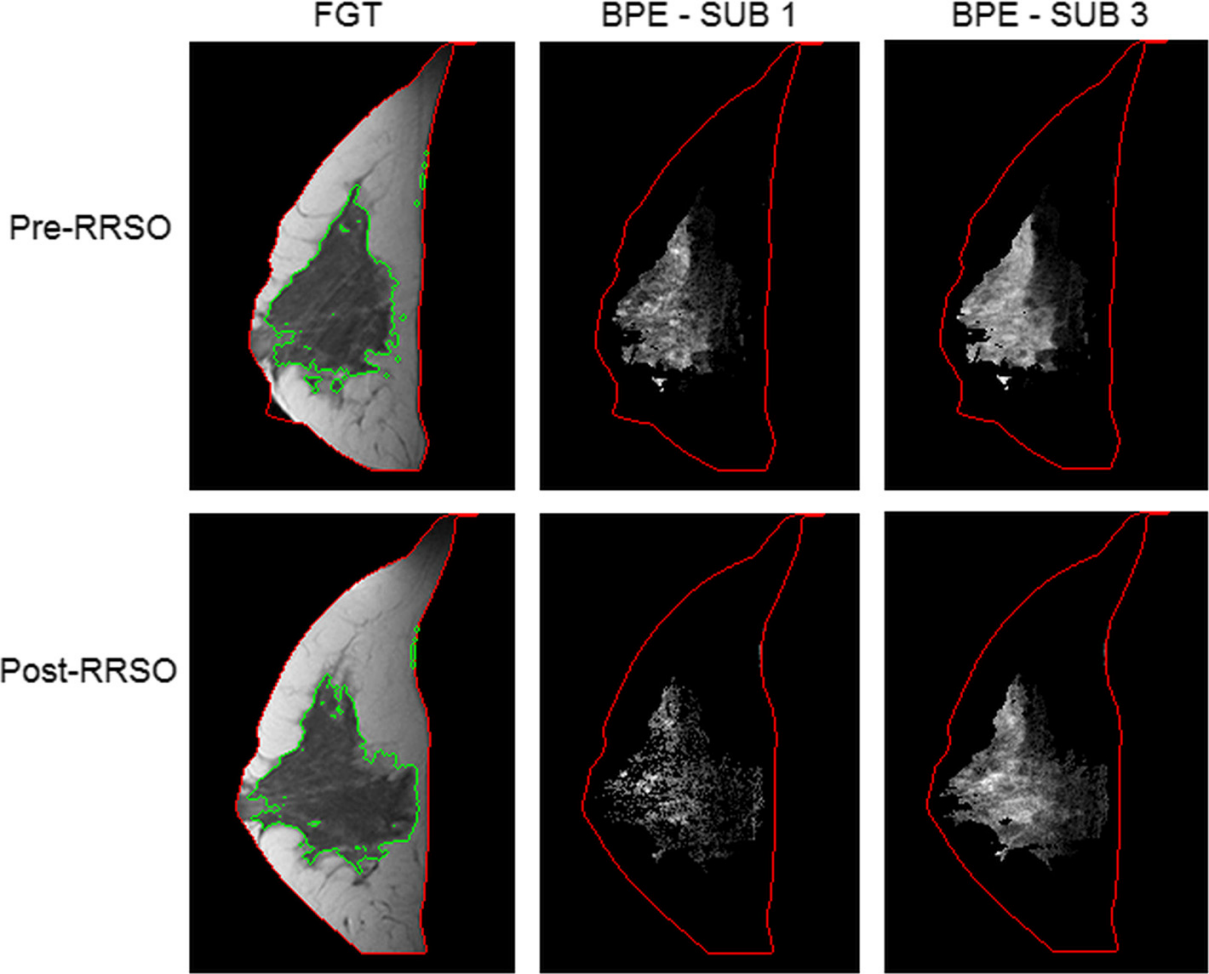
Representative examples of fibroglandular tissue (FGT) and background parenchymal enhancement (BPE) from a magnetic resonance imaging (MRI) slice obtained pre-RRSO and post-RRSO in a woman who developed breast cancer after undergoing risk-reducing salpingo-oophorectomy (RRSO). FGT is circumscribed by *green contours*. BPE is estimated in both the first and third subtraction series (SUB 1 and SUB 3, respectively). This 36-year-old (at time of RRSO) woman had her pre-RRSO MRI at 1 month before RRSO and her post-RRSO MRI at 6 months after RRSO. Breast cancer (ductal carcinoma in situ) was diagnosed in her right breast 6 years after RRSO, and her final pathologic examination showed in situ carcinoma with 2 foci of microinvasion (<0.1 cm). This example shows no decrease of BPE and FGT after RRSO. The volumetric pre-RRSO BPE % values were 10.7 % (SUB 1) and 10.6 % (SUB 3) and after RRSO, and BPE % values were 7.1 % (SUB 1) and 8.5 % (SUB 3)
